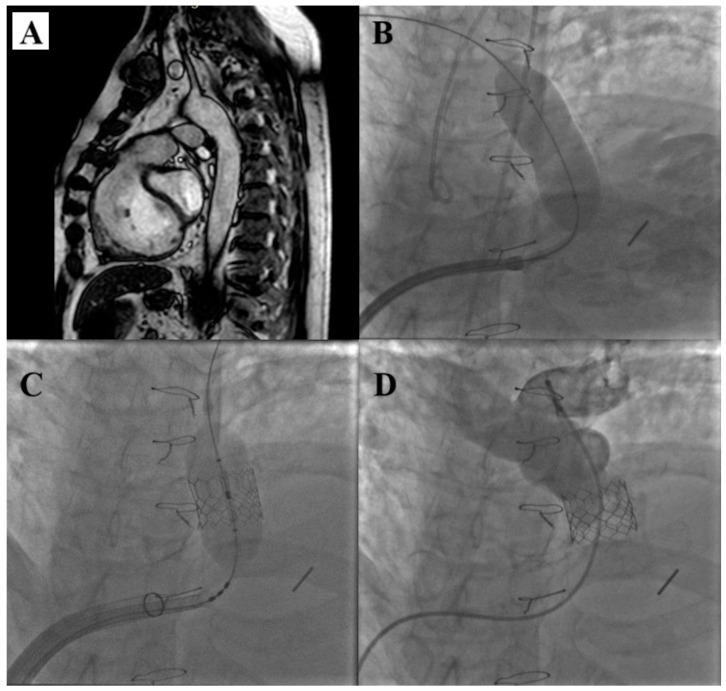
Direct Edwards Sapien 3 implantation. Cardiac MRI highlights a severe pulmonary regurgitation with a significant right ventricle dilatation (A). RVOT balloon interrogation with an occlusive semi-compliant 28 mm balloon (B). A direct implantation of an Edwards Sapien 3 29 mm (C). Pulmonary artery injection demonstrates a well-deployed continent Edwards Sapien 3 (D).
